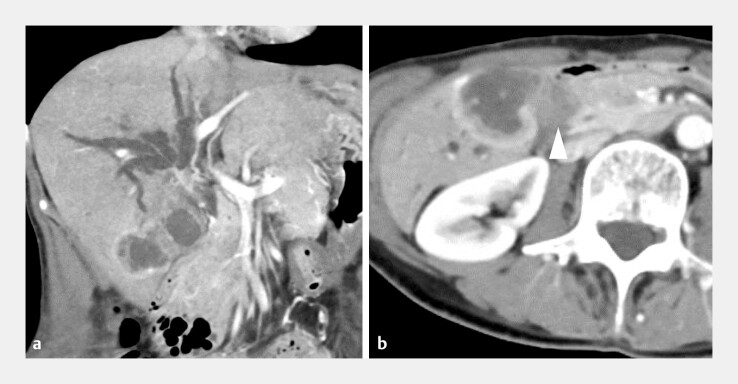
Computed tomographic images before biliary drainage.
